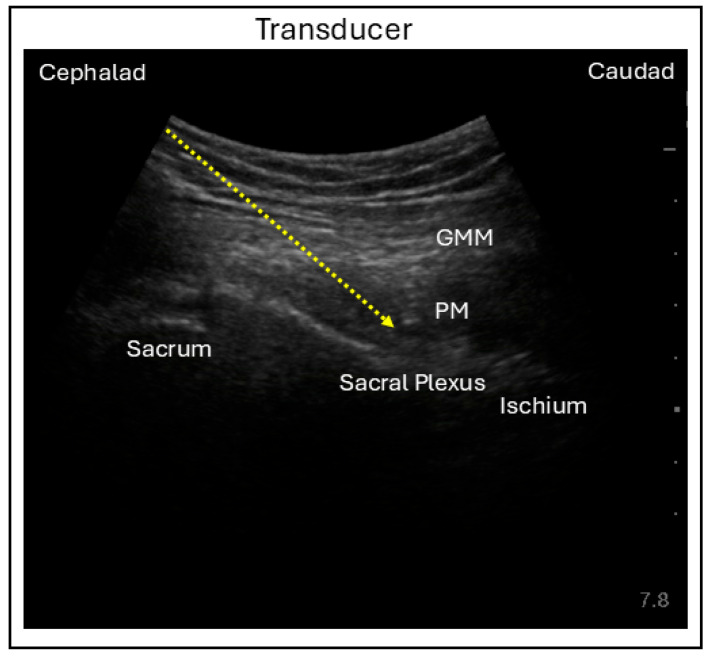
Ultrasound image demonstrating the in-plane lateral parasacral sciatic nerve block technique. GMM = Gluteus maximus muscle; PM = Pyriformis muscle; Dashed line = Needle trajectory.
